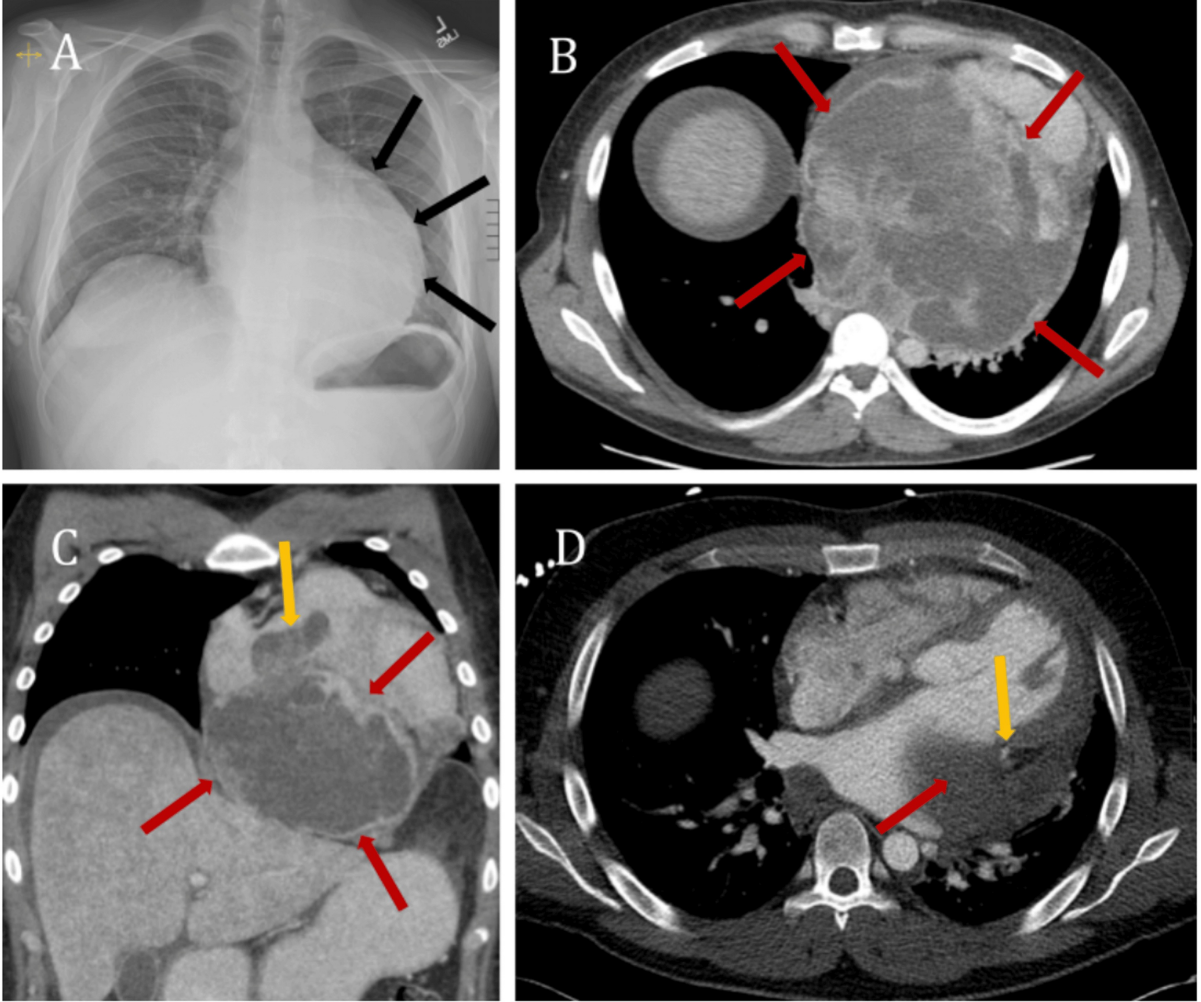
(A) Chest radiograph (PA view) with enlarged cardiac silhouette (black arrows in A). (B) Axial CECT of the lower chest with a large heterogeneously enhancing necrotic mass along the posterior inferior aspect of the heart, originating from the pericardium (red arrows in B). (C) Coronal CECT of the chest with a large pericardial mass (red arrows in C) infiltrating into the right atrium and right ventricle (yellow arrow in C). (D) Axial CECT of the mid chest with the partially visualized pericardial mass (red arrow in D), completely encasing the proximal left circumflex coronary artery (yellow arrow in D). The mid and distal left circumflex coronary artery (not seen in this section) was completely obliterated. PA: posteroanterior, CECT: contrast-enhanced computed tomography
